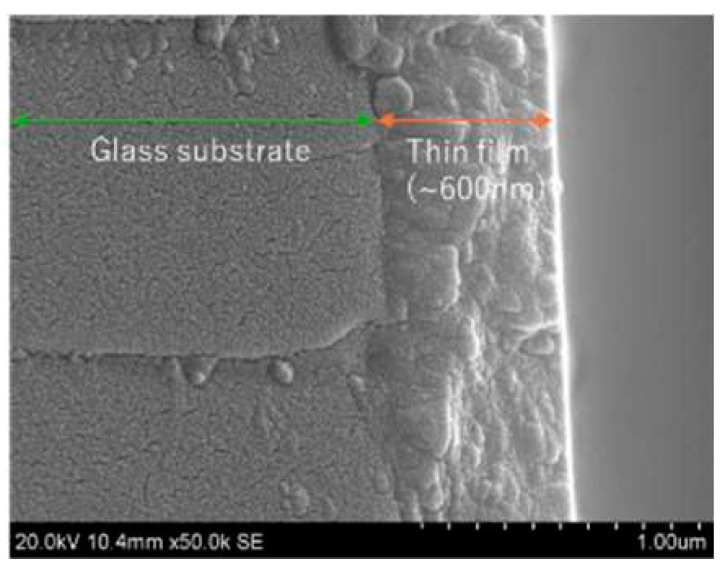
Cross section of the thin film as seen through the SEM ($n_{Al}$ = 2.1 at.%). The white parts indicate a larger amount of free electrons. The quartz glass was cleaned by ultrasonic washing machine in acetone before the formation of AZO films.
